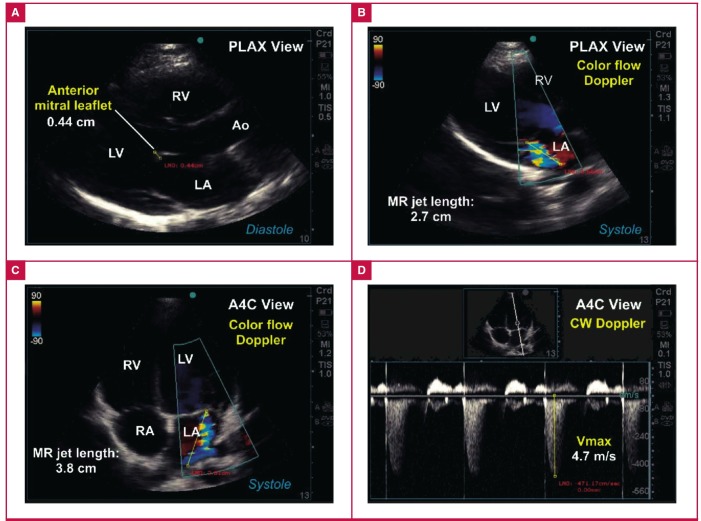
Example of definite RHD consistent with WHF 2012 criteria. A. Parasternal long-axis (PLAX) view showing thickened anterior mitral leaflet exceeding 3 mm in young adult study subject. B. Colour-flow Doppler interrogation of the mitral valve shows an eccentric jet of mitral regurgitation (MR) with jet length exceeding 2 cm. C. Colour-flow Doppler interrogation of the mitral valve using the apical four-chamber (A4C) view shows eccentric jet of mitral regurgitation with jet length measuring 3.8 cm. D. Continuous-wave (CW) Doppler interrogation of the MR jet showed maximum velocities exceeding 4.7 m/s.
